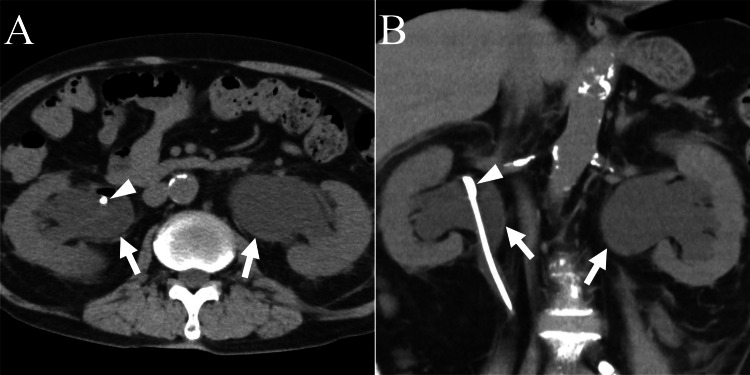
Abdominal computed tomography (CT). Axial (A) and coronal (B) CT images showing bilateral hydronephrosis (arrows). A double-J stent (arrowhead) is observed in the right renal pelvis.
